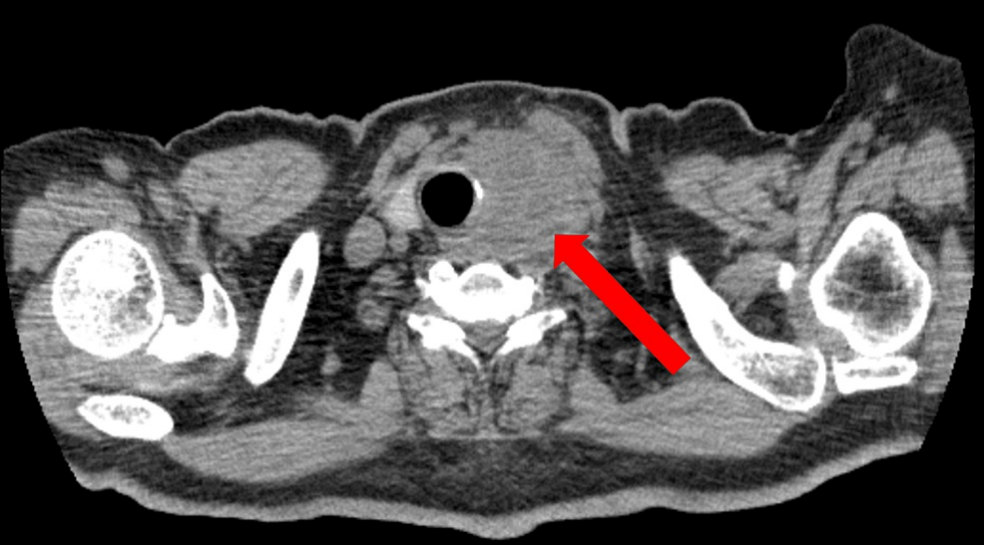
Neck CT Neck CT showing enlargement of the left lobe of the thyroid gland (red arrow).
